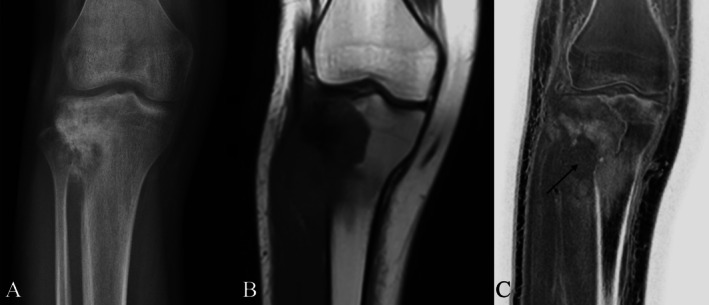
Radiograph of the knee (A), Coronal T1 MRI (B) and FRACTURE MRI of the knee (C) in a patient with proximal tibial osteogenic sarcoma. Note the extraosseous soft tissue better appreciated on T1 and FRACTURE MRI (arrow). Surrounding bone marrow edema is also seen on T1 as hypointensity, which is represented by sclerosis on FRACTURE MRI.
